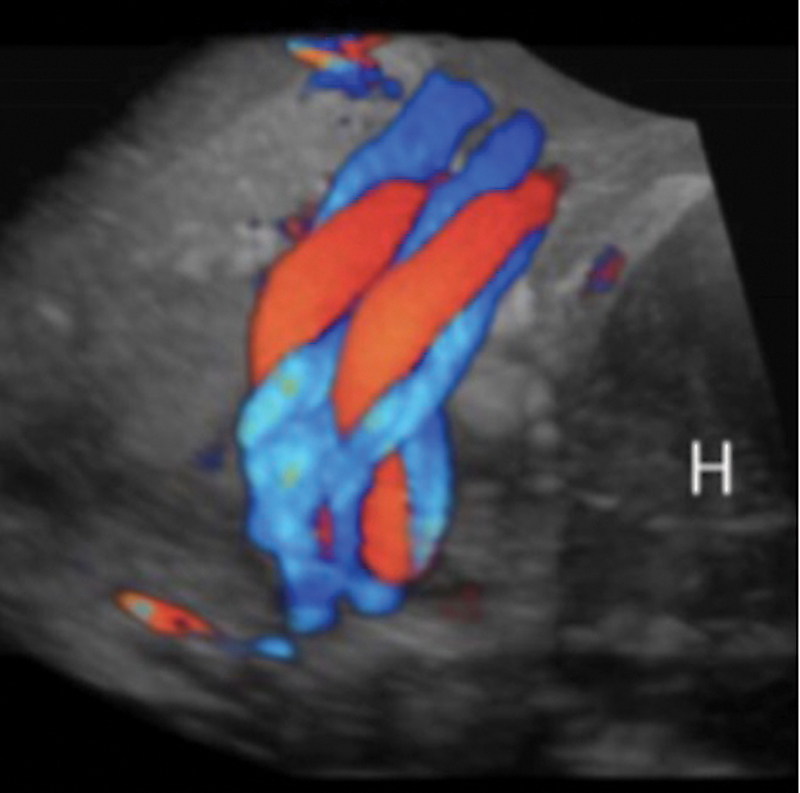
Ultrasound imaging showing multiple nuchal cord loops.
